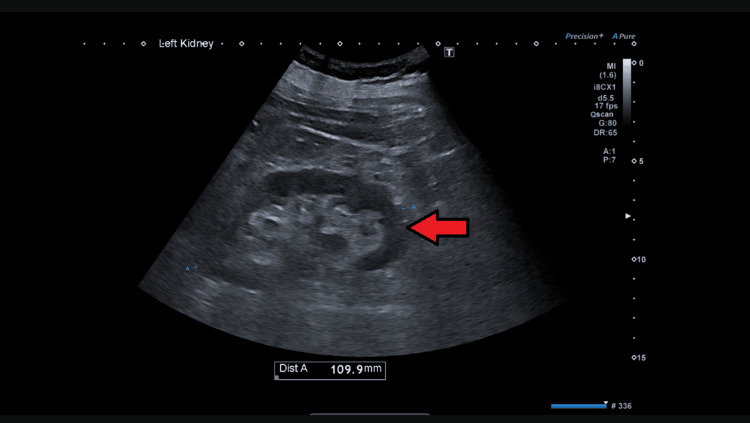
Ultrasound of the kidney, ureter, and bladder There was no significant hydronephrosis seen in the ultrasound. The left kidney measured around 10.9 cm. The red arrowhead indicates preserved corticomedullary differentiations.
